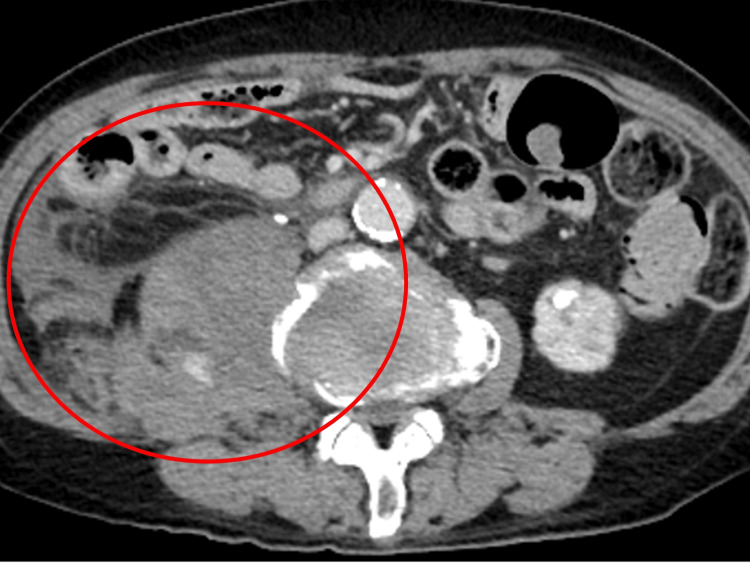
Contrast-enhanced computed tomography revealing bleeding within the right iliopsoas muscle with suspected continuous bleeding (red circle) Contrast-enhanced computed tomography demonstrated a heterogeneous hyperdense hematoma within the right iliopsoas muscle, measuring approximately 66 × 58 mm. Areas of active contrast extravasation were visible, indicating ongoing bleeding. The hematoma showed irregular margins without rim enhancement, consistent with acute intramuscular hemorrhage.
